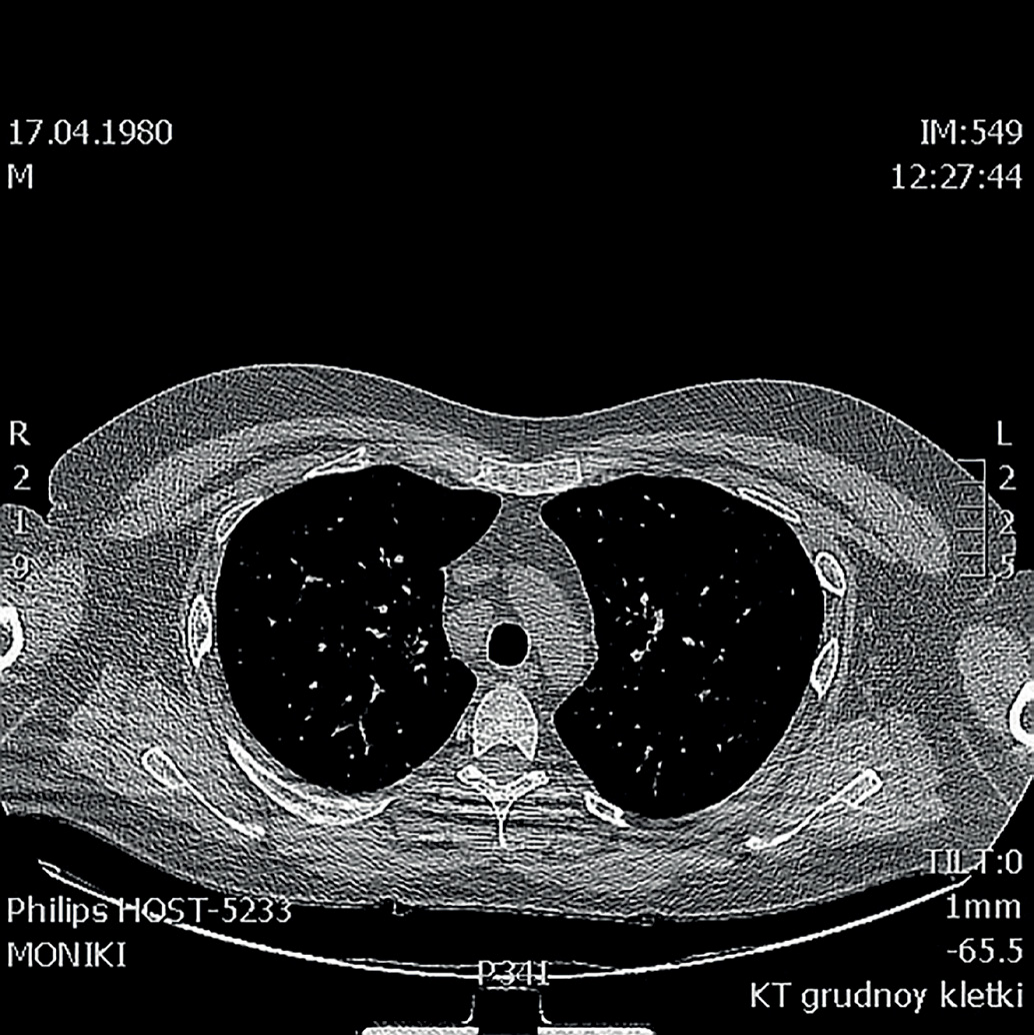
Рисунок 3. КТ органов грудной клетки от 2018г.(Немногочисленные мелкоочаговые тени лимфогенного распределения, лучше визуализируются в проекции междолевой плевры правого легкого). Figure 3. CT scan of the chest from 2018(A few small focal shadows of the lymphogenous distribution are better visualized in the projection of the interlobar pleura of the right lung).
